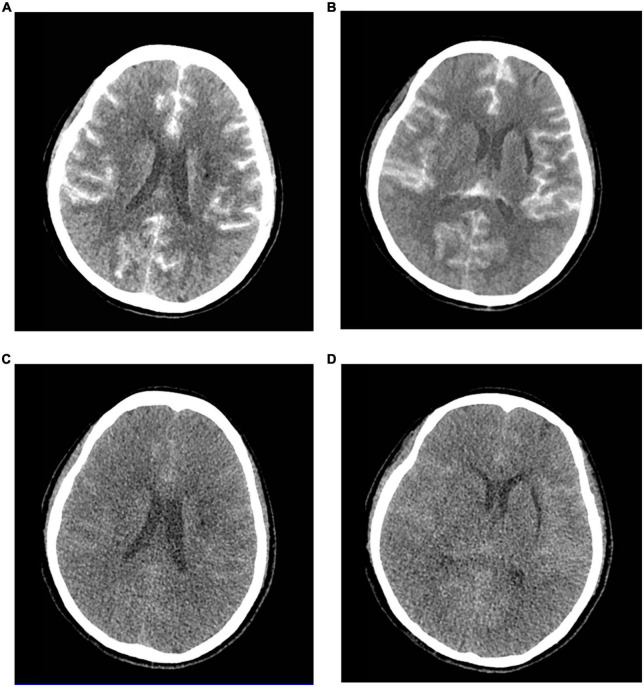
Contrast-induced encephalopathy in brain computed tomography (CT) scans. (A,B) Emergency brain CT 5 h after the procedure showed diffuse contrast enhancement in the brain parenchyma and subarachnoid space with mild global brain edema and the softening foci in the left basal ganglia-insular area. (C,D) Brain CT 2 days after the procedure indicated diffuse enhancement disappeared, and the brain parenchyma was diffusely swollen and the lateral ventricle was slightly more compressed.
